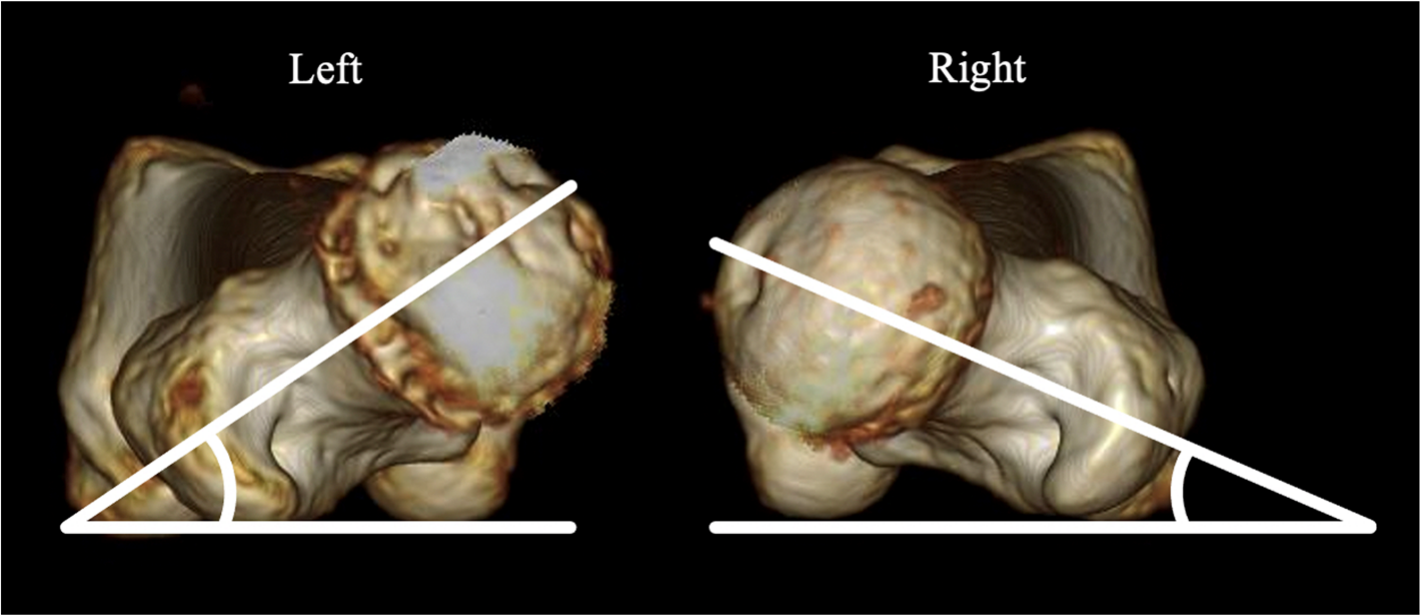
Plain 3D-CT. The anteversion angle of the femoral necks (left/right) was 30/20 degrees. Furthermore, osteophyte formation on the anterior femoral head is present
